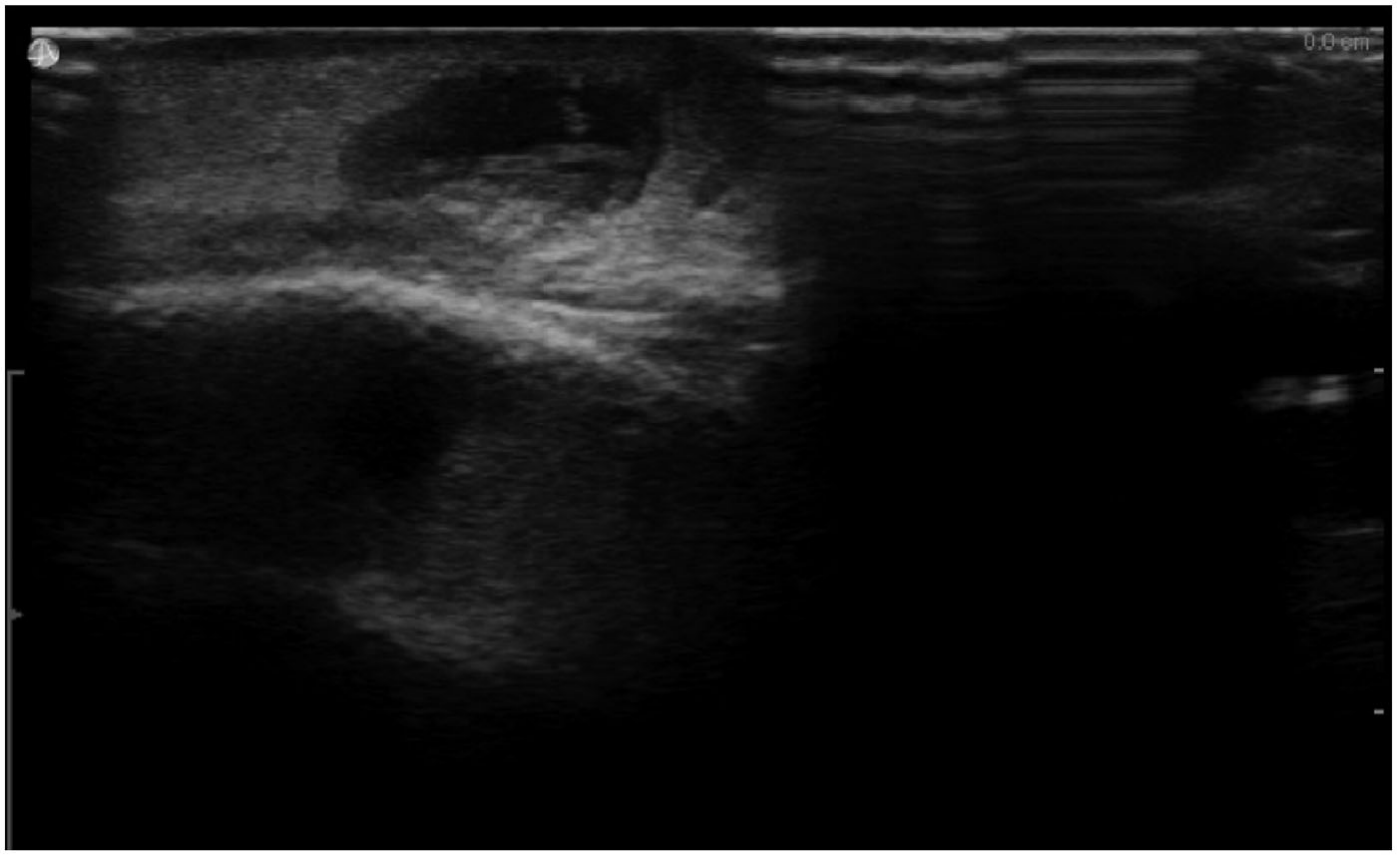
An ultrasound image of the motile nematode.
